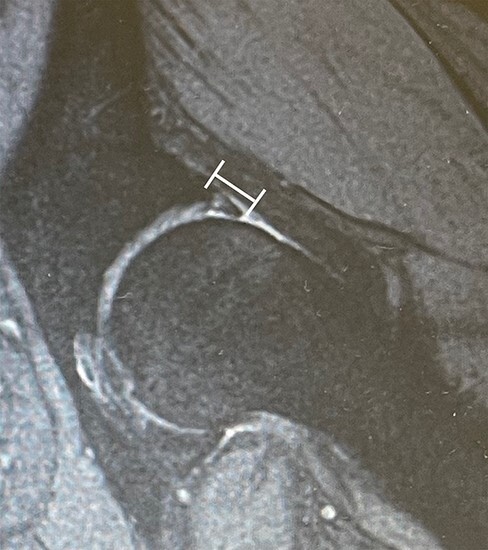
Measurement to labral parameter. Measurement as the distance from the acetabular rim to the edge of the labral length at each slice of the radial MRI scan (white line).
